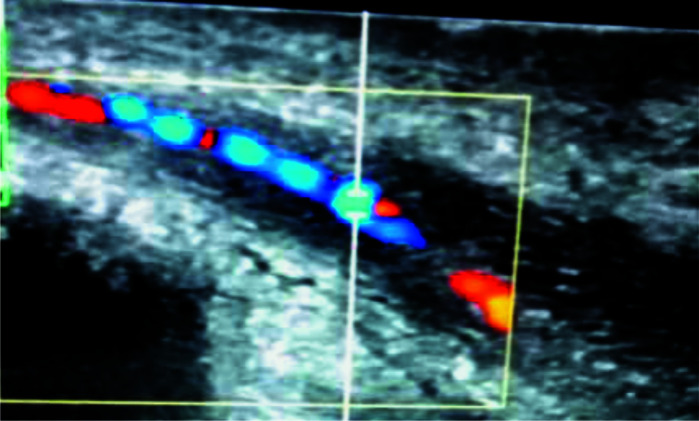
Left CA colour Doppler showing multiple areas of narrowing and dilatations, giving beaded appearance
